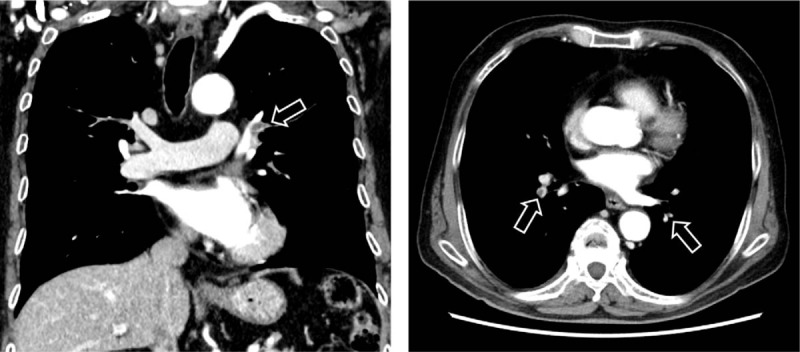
Chest computed tomography scan on admission showing a contrast deficit in the bilateral pulmonary artery (arrow).
